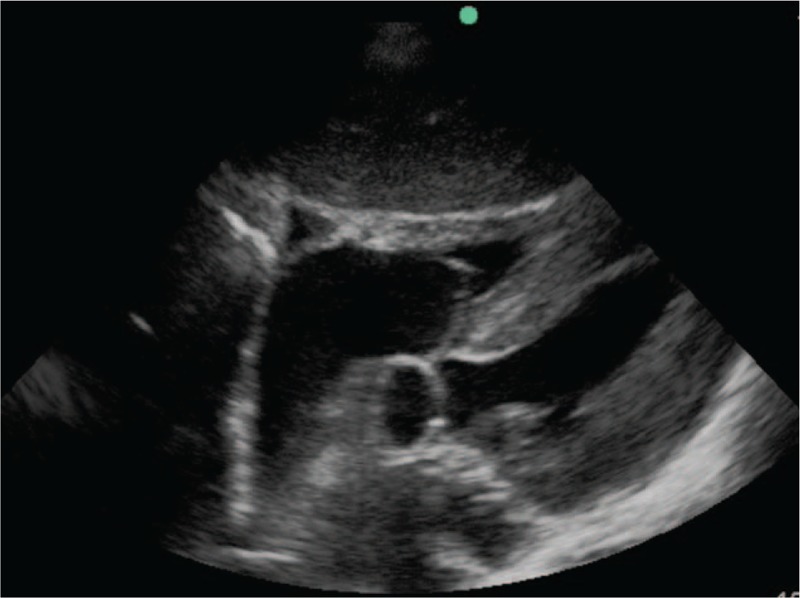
Echocardiographic examination at ICU admission showed an enlarged left atrium and left ventricle, and mild pericardial effusion. ICU = intensive care unit.
